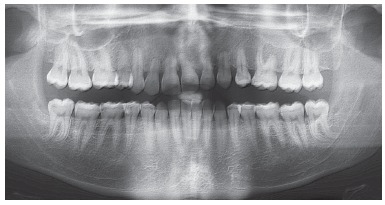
Baseline panoramic radiograph.
